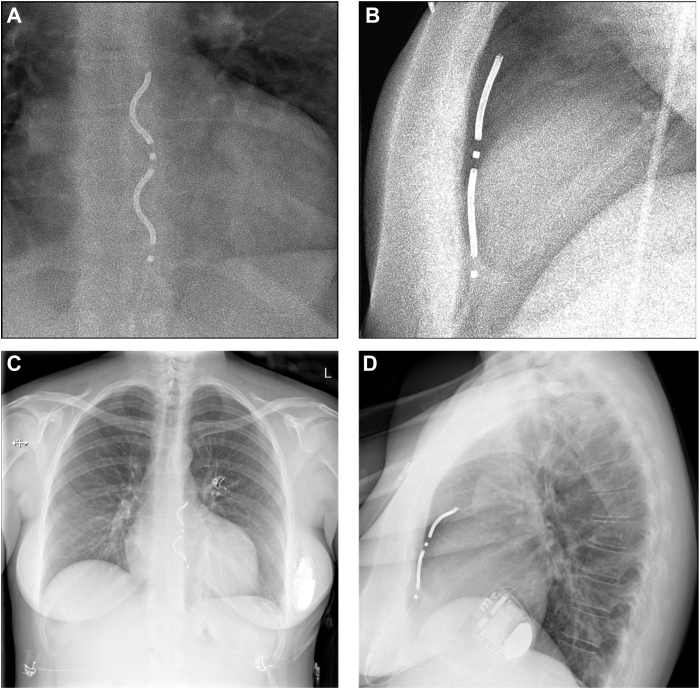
Extravascular implantable cardioverter-defibrillator (EV-ICD) lead position in case 1. EV-ICD lead position during the implantation from the anteroposterior (A) and the left anterior oblique 90-degrees (B) views. Post-implantation chest radiograph shows unchangeable lead position in the anteroposterior view (C), but posterior tilting in the lateral view (D).
